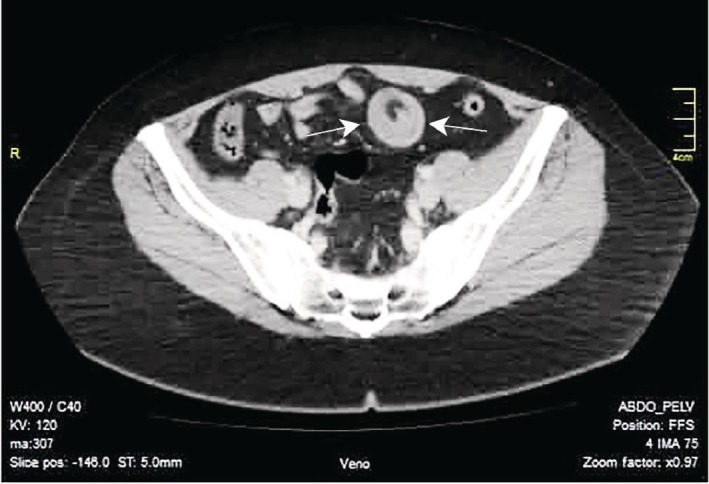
Axial contrast‐enhanced CT image demonstrating small bowel intussusception with a characteristic bowel‐within‐bowel configuration (arrows) and an intraluminal lead point, without signs of ischemia or perforation.
